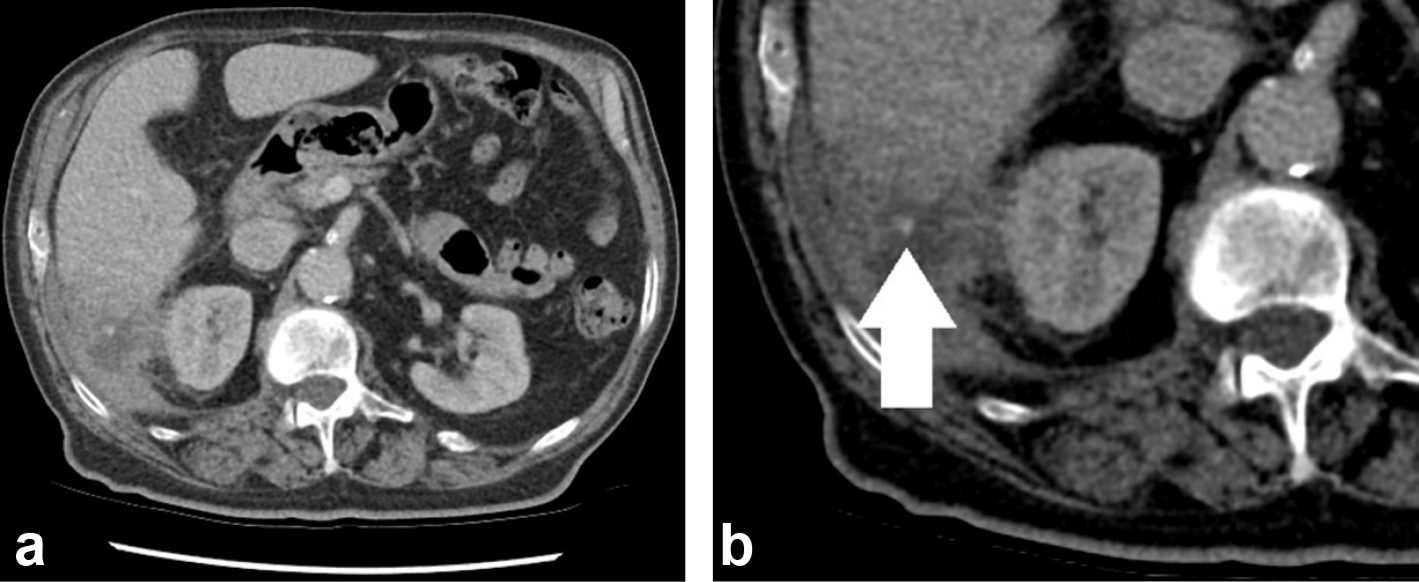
CT following intravenous contrast demonstrating the (a) perihepatic
